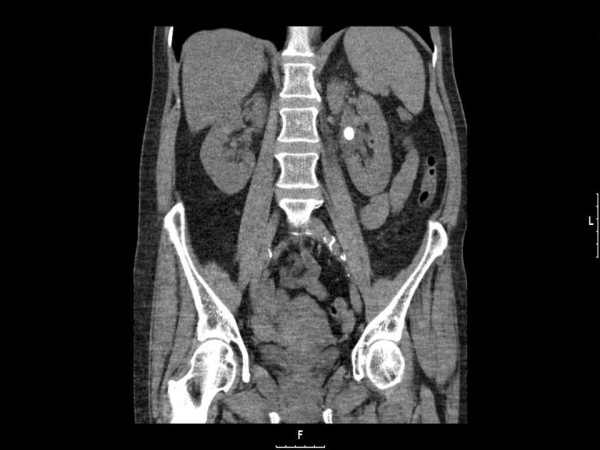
Computed tomography of the kidneys, ureter and bladder, prior to percutaneous nephrolithotomy. Note a two centimetre stone located at the left renal pelvis.
